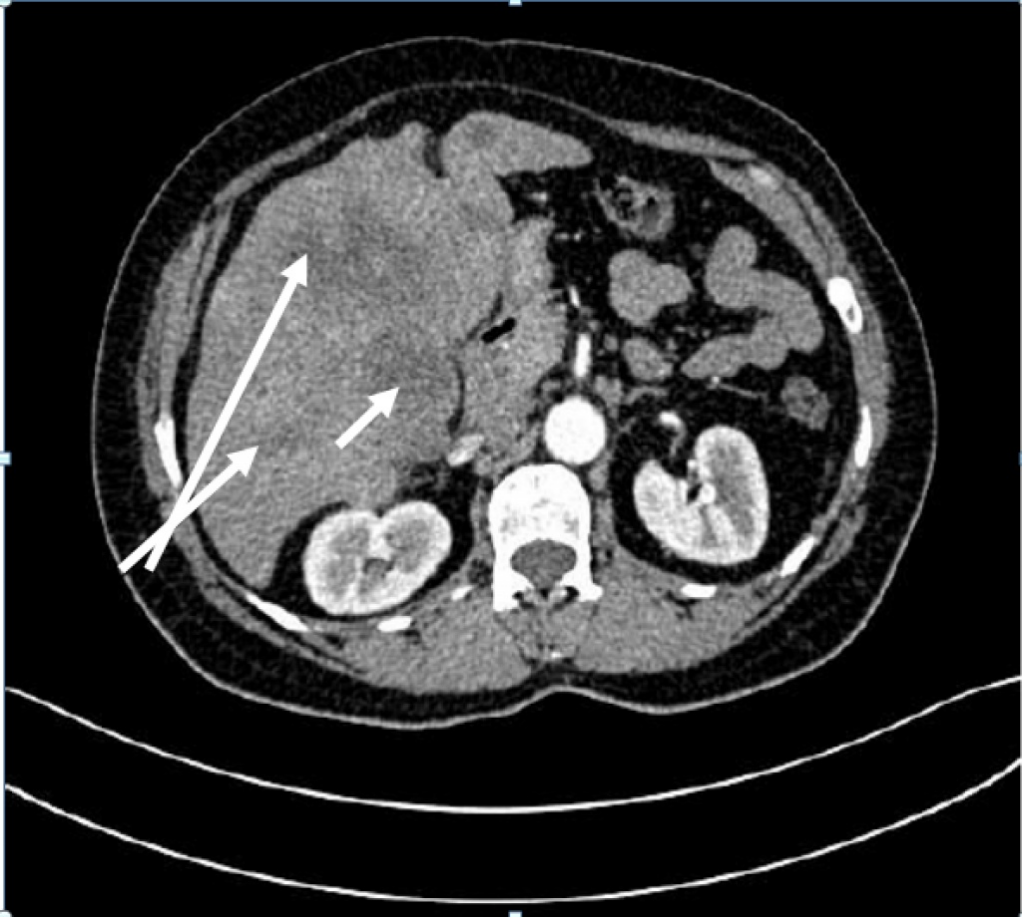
Abdominal computed tomography: 10.5 × 9 cm mass replacing the gallbladder with liver metastases.
